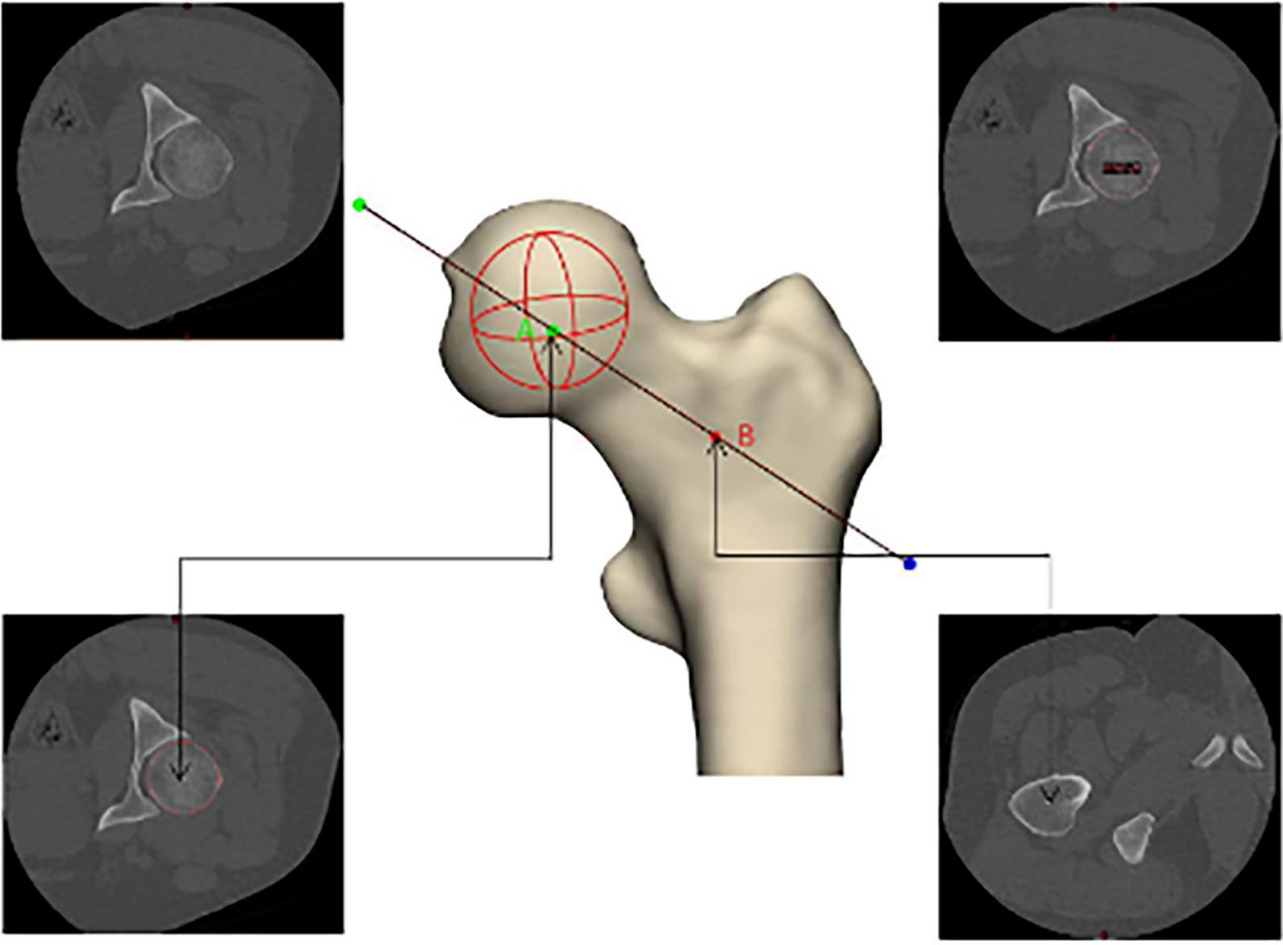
Murphy’s method identifies the axis of the femoral neck. The top left is a random CT plan of the femoral head; The figure on the upper right shows the diameter of the femoral head on the CT plane. The figure at the lower left is the maximum diameter of the femoral head in all CT planes (the center of the circle in CT plane is marked as A, that is, the center of the femoral head in the fitting sphere). The lower right figure is a CT plan of the base of the neck of the femur (the upper margin of the lesser trochanter, roughly triangular, and the center of the triangle is B, the center of the base of the neck of the femur)
